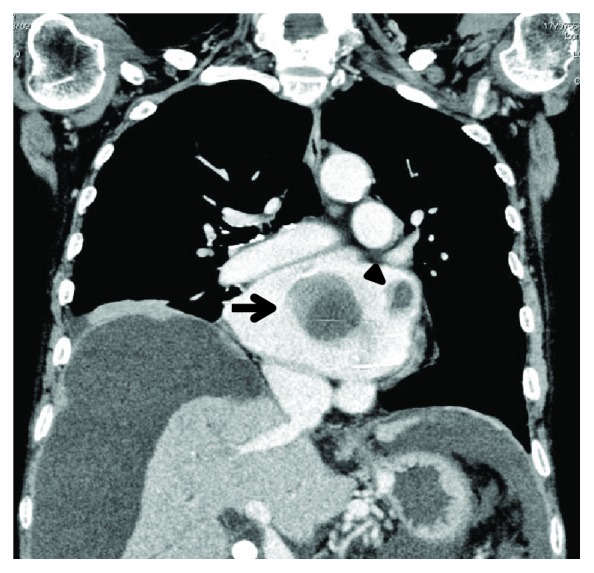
Computed tomography scan of the heart showing the free-floating ball-shaped thrombus (arrow) and appendage thrombus (arrow head). They look blurred due to movement within the left atrium.
